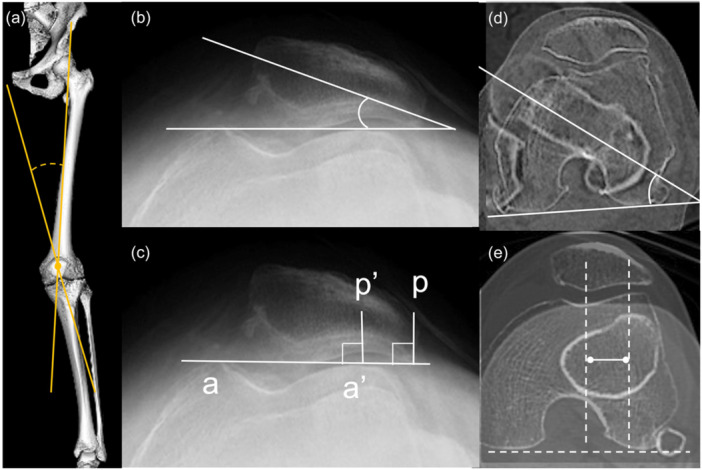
Radiographic assessments. (a) Radiological quadriceps angle (rQ angle) was measured as the acute angle between the line from the anterior superior iliac spine to the centre of the patella and the line from the tibial tuberosity to the centre of the patella. (b) Tilting angle was defined as the angle between the line intersecting the widest bony structure of the patella and the line tangentially passing the anterior surface of the femoral condyles. (c) Lateral shift ratio (LSR) was defined as the ratio of the distances pp′/aa′. aa′ is defined as the distance between the summits of the medial and lateral femoral condyles. pp′ is defined as the distance between the summit of the lateral femoral condyle and the point where a line from the lateral edge of the patella perpendicular to the line that passes through the summits of the femoral condyles crosses that line. (d) Femoral anteversion was measured as torsional angle of the femur between the line drawn parallel to the posterior femoral condyles and the line drawn through the centre of the femoral neck on the oblique axial CT. (e) Tibial tuberosity–trochlear groove (TT‐TG) distance was measured from two superimposed CT slices, one through the floor of the groove where the intercondylar notch has a Norman arch shape, and the other through the middle of the tibial tubercle. CT, computed tomography.
